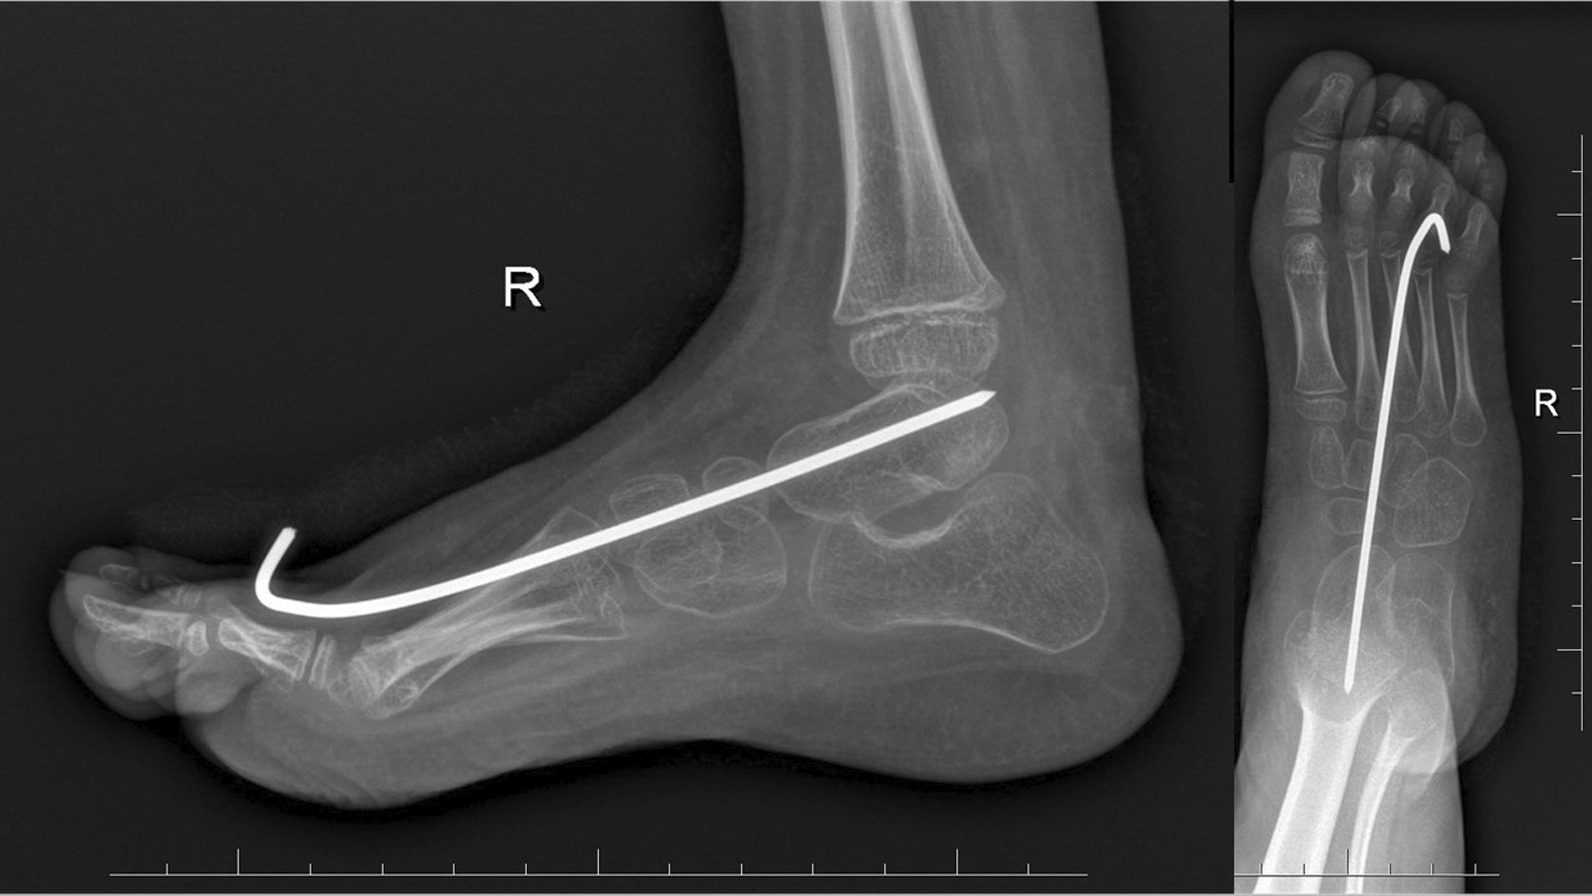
Case 2 AP and L X-ray after surgery
